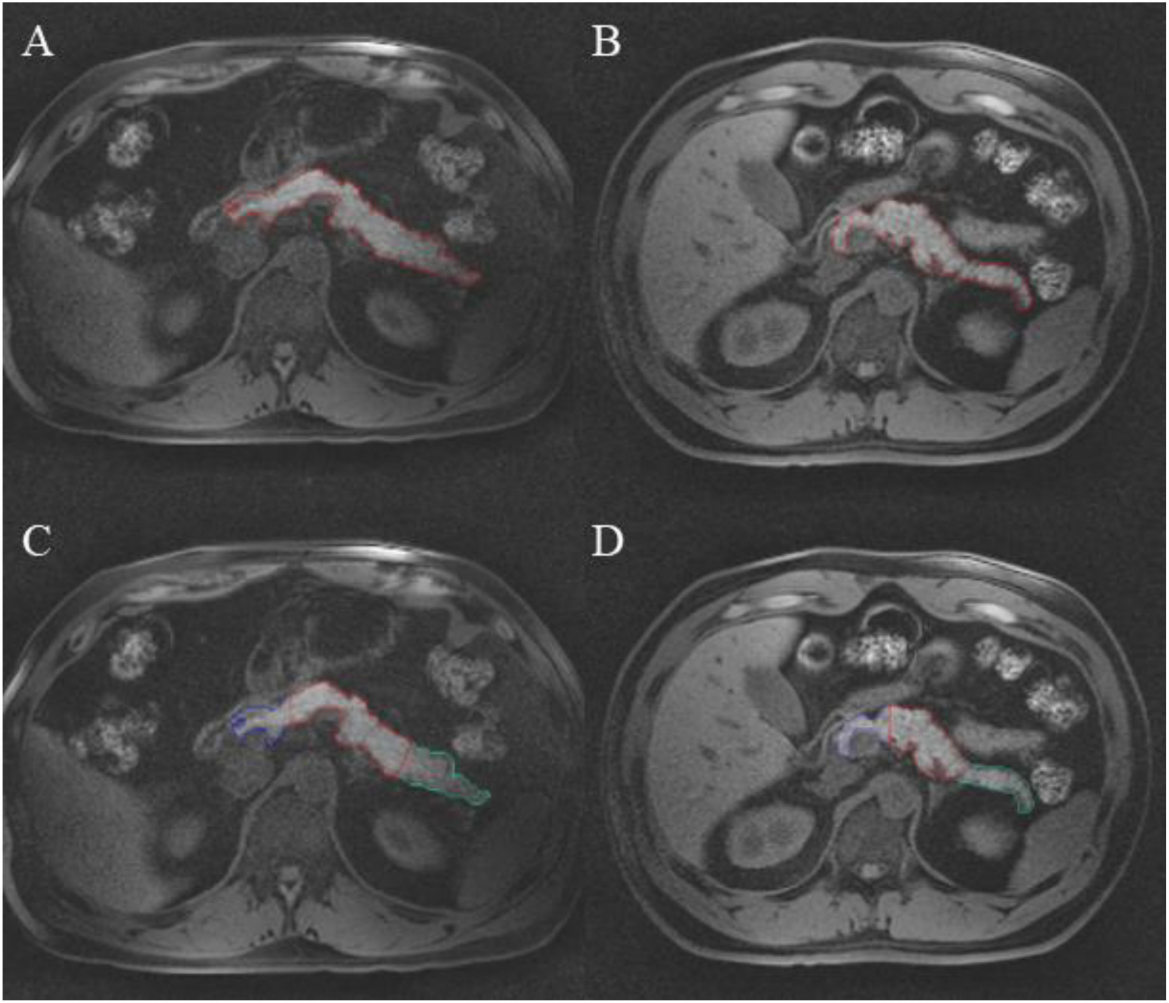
Representative axial pancreas MR images of whole pancreas region of interest in a (A) healthy obese volunteer and (B) individual with type 2 diabetes. Subdivision of pancreas regions of interest for head (blue), body (red), and tail (green) in (C) a healthy obese volunteer and (D) an individual with T2D. T2D, type 2 diabetes.
